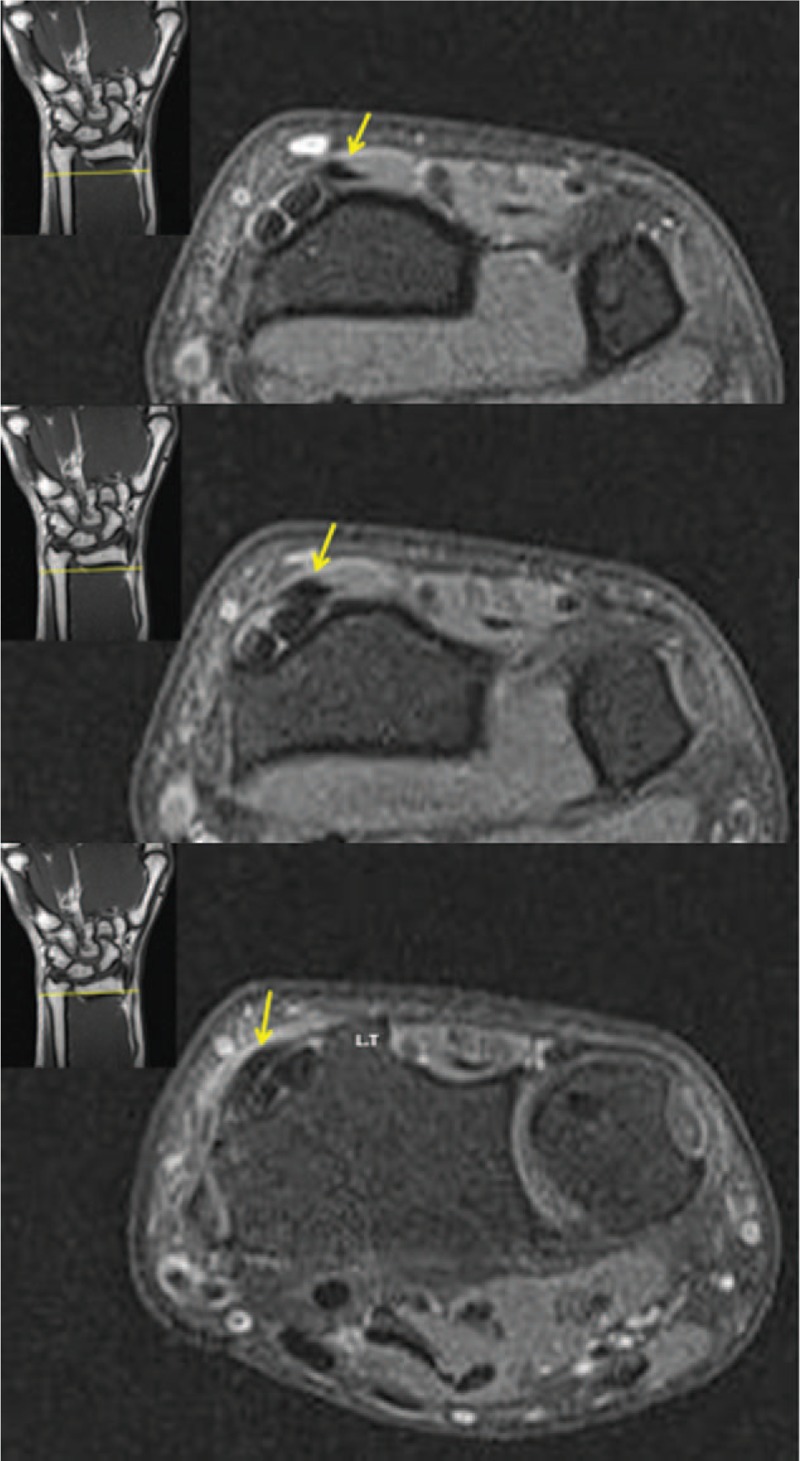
Coronal (A) and axial (B) fat-suppressed T2-weighted images at the left wrist level showing the oblique course of EPL tendon, which originated in the ulnar side of the forearm and extended to radial styloid at the radial and proximal sites of Lister's tubercle. (Arrows indicate the EPL tendon, LT: Lister's tubercle). EPL = extensor pollicis longus.
